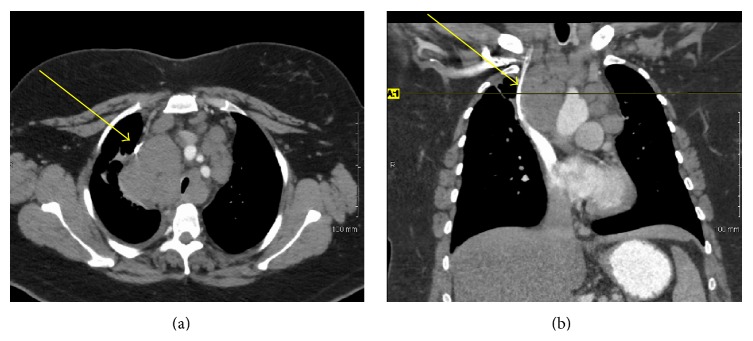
Axial (a) and coronal (b) chest CT images showing mediastinal lymphadenopathy compressing the SVC (arrows) and trachea. The axial image in (a) is at the level of the horizontal line noted in (b).
